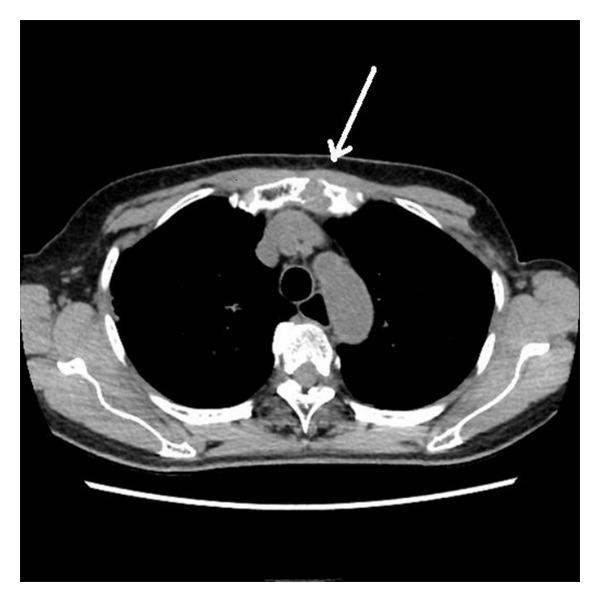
Computed tomography scans of the mass. The arrow indicates the sternal metastasis.
